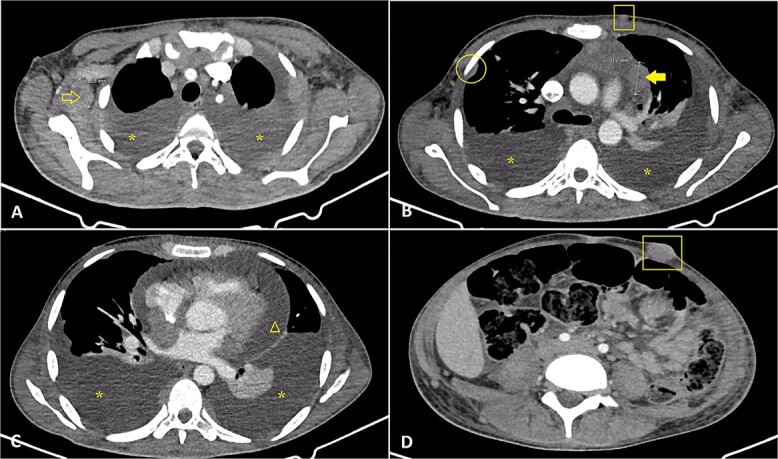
Selected axial cuts of a chest and abdomen CT scan were done for the patient, showing moderate bilateral pleural effusions (Asterisks) (A, B, and C) and pericardial effusion (Triangular) (C). In addition, pleural-based nodules are noted (Circle) (B), associated with multiple enlarged mediastinal (Solid arrow) and bilateral axillary (Open arrow) lymph nodes (A, B). A few subcutaneous enhancing nodules are also noted (Squares) (B, D). These features are related to widespread metastatic disease.
